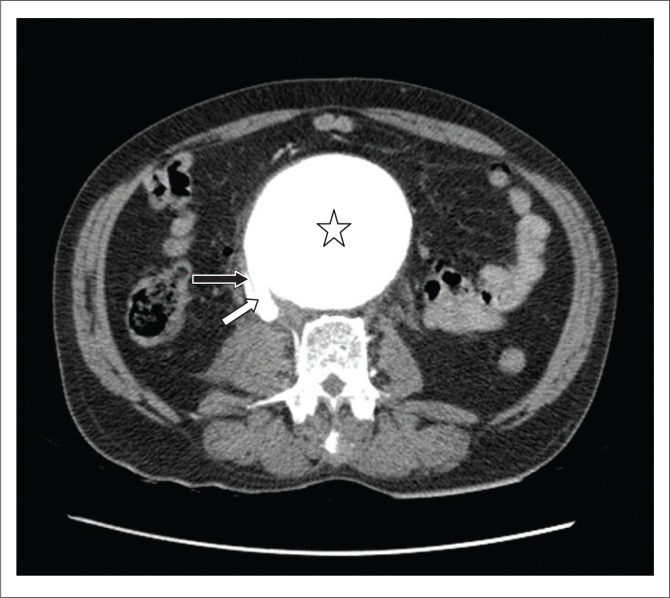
Axial contrast-enhanced computed tomography showing a large infrarenal aneurysm (star) having a fistulous communication (white diagonal arrow) on its right posterolateral wall with inferior vena cava (black arrow).
